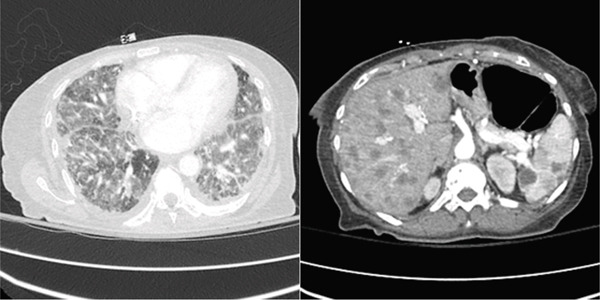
Key images from the repeat CT of the chest, abdomen, and pelvis demonstrating worsening lymphangitic spread of the patient′s EHE.
